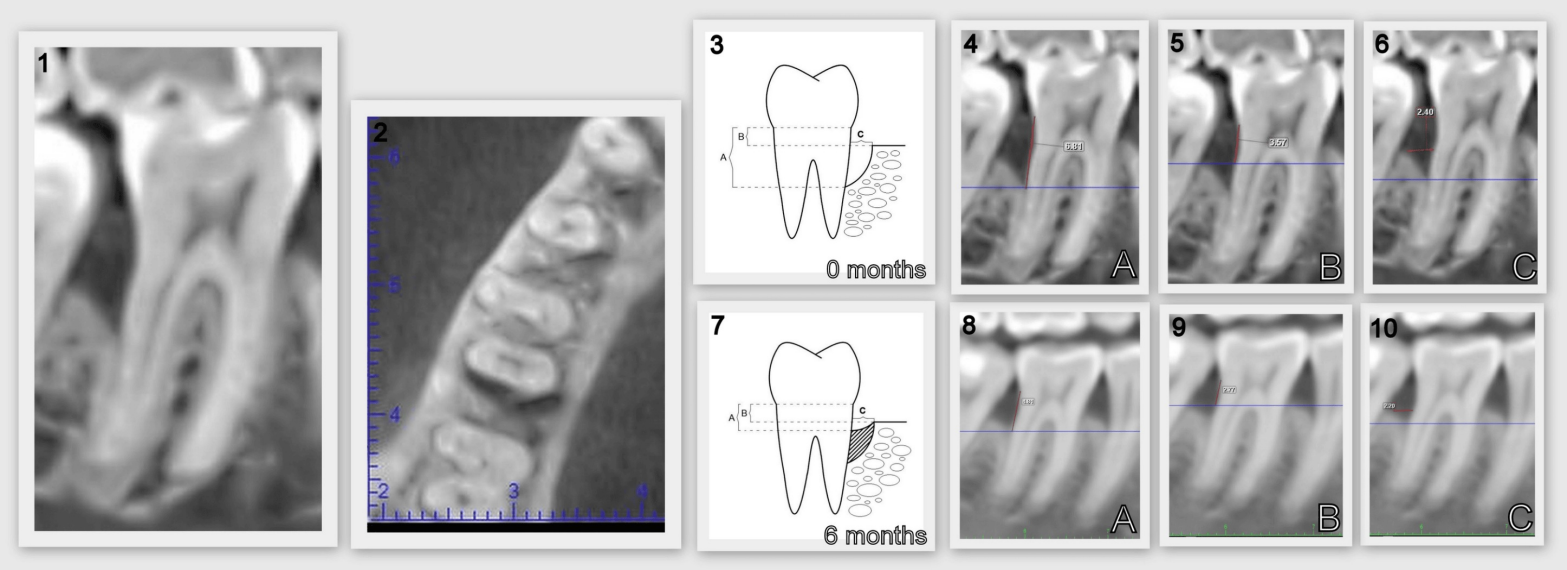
CBCT parameters (1) Coronal plane of the vertical defect on CBCT. (2) Transversal plane of the vertical defect on CBCT. (3) CBCT parameters studied before regenerative therapy (authors' own image). (4) Parameter A (0 months). (5) Parameter B (0 months). (6) Parameter C (0 months). (7) CBCT parameters studied 6 months after regenerative therapy (authors' own image). (8) Parameter A (6 months). (9) Parameter B (6 months). (10) Parameter C (6 months) CBCT: cone-beam computed tomography
